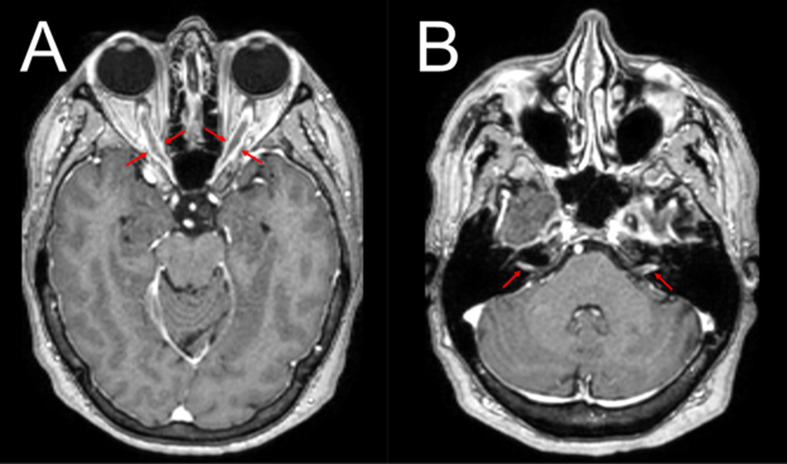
A. Orbit MRI (post-contrast axial T1-weighted) revealed
